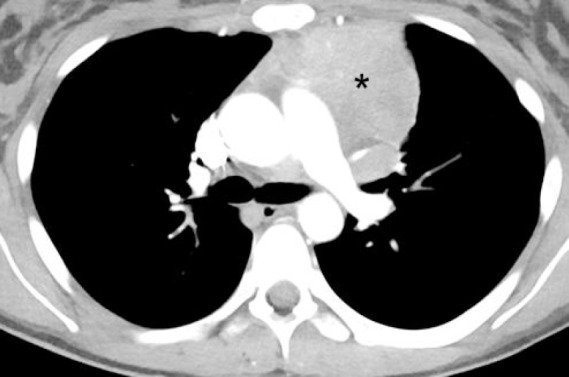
Contrast enhanced CT of the chest obtained at the level of tracheal bifurcation shows a large mediastinal mass (asterisk) anterior and to the left of the main pulmonary trunk in a patient with non-Hodgkin lymphoma
